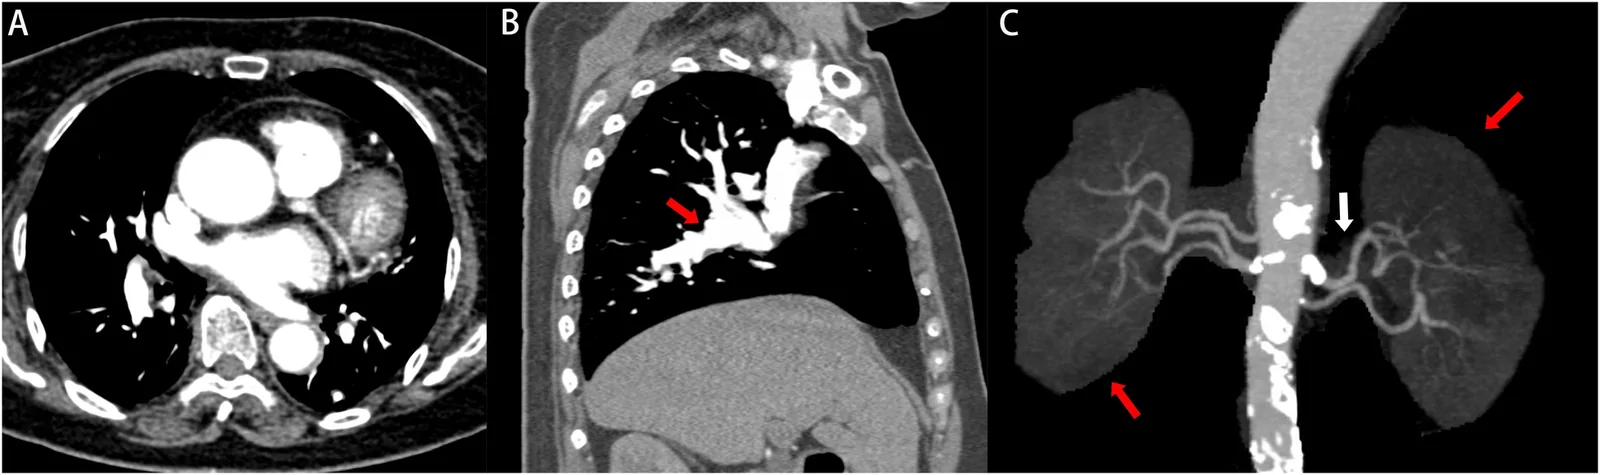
(A) pulmonary CTA showed complete resolution of emboli in the bilateral lower lobe pulmonary arteries, with restoration of contrast opacification and improved visualization of distal segmental pulmonary arterial branches. (B) Oblique sagittal reconstruction of pulmonary CTA confirmed successful thrombus clearance and restoration of patency in the right pulmonary artery (red arrow). (C) Mesenteric CTA demonstrated spontaneous recanalization of the previously embolized left main renal artery (white arrow) and the right accessory renal artery, along with markedly improved renal parenchymal perfusion and near-complete resolution of bilateral renal infarct areas (red arrows).
